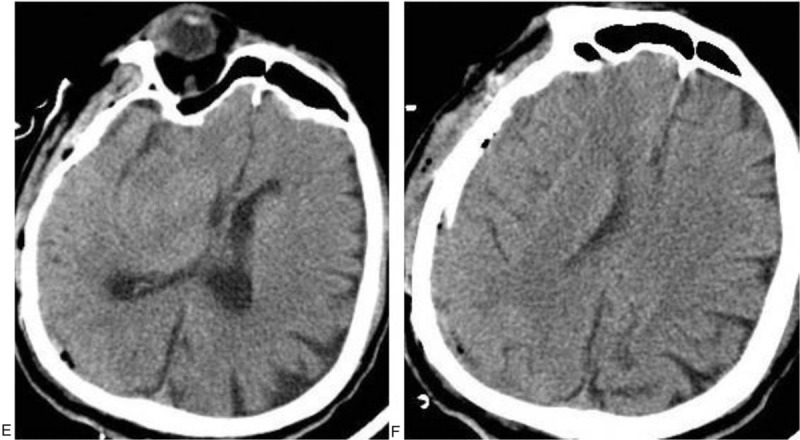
Postoperative reexamination by CT showed that the slightly higher bands below the right frontotemporal top plates and the low-density shape had disappeared, the right brain tissues were relieved from compression, and the sulcus had reappeared. The median line had returned to a normal position.
